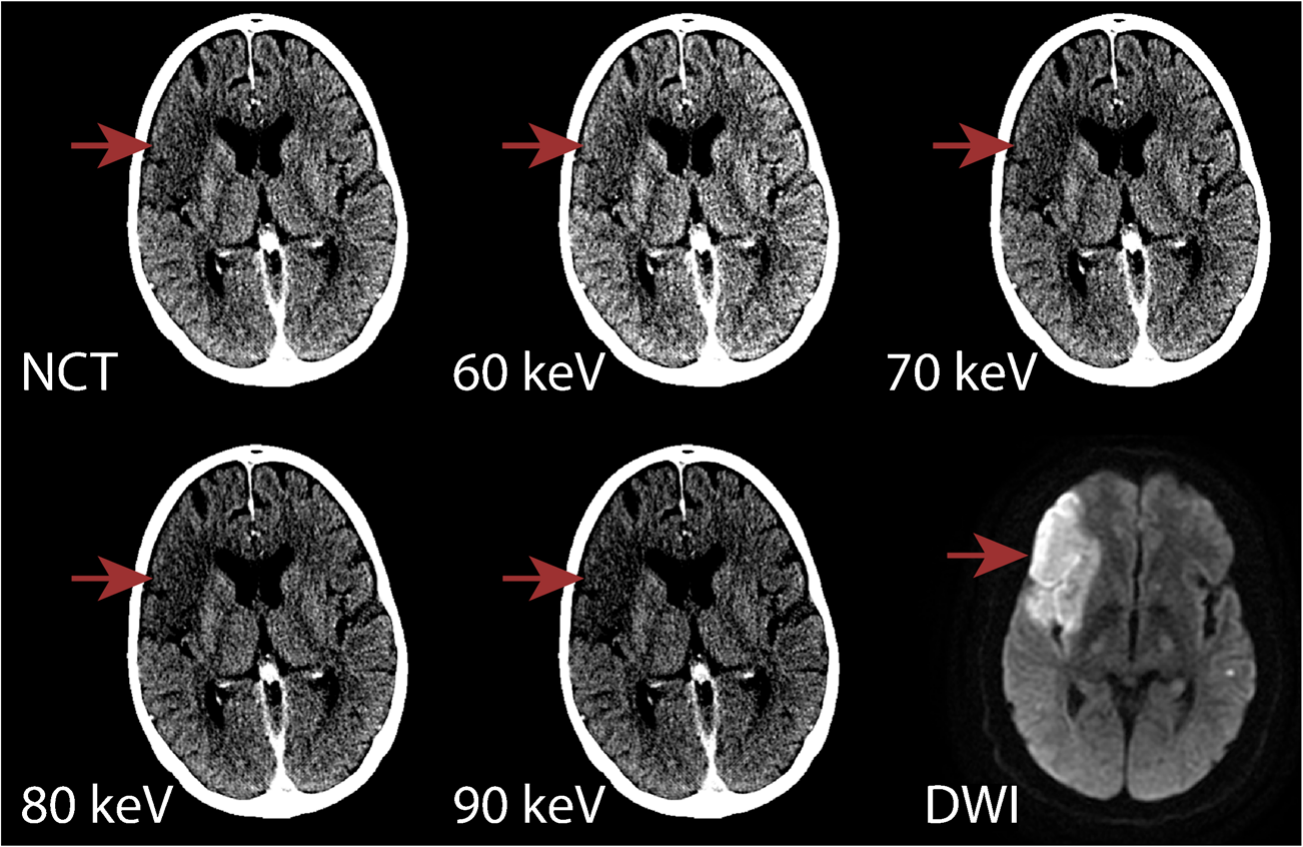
Example case of a patient demonstrating the increased conspicuity of an acute infarct on the 80 and 90 keV VMI in comparison to NCT, 60 and 70 keV and corresponding diffusion restriction on subsequent MRI DWI confirmed the territory of infarct. Red arrows indicate the outlining of the infarcted area. The CT reconstructions have the same WL/WW, 40/30
